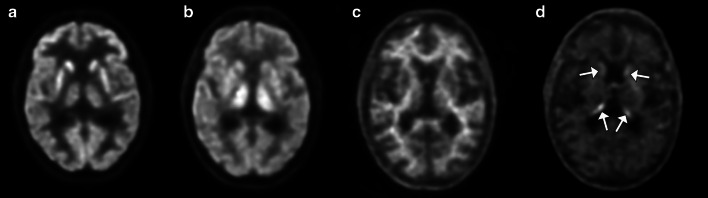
Normal patterns of cerebral uptake across multiple radiotracers. Fluorodeoxyglucose PET/CT demonstrates high-grade uptake in the cortical and deep gray matter which should be greater than cerebellum (a). Cerebral blood flow imaging with early-frame or dynamic amyloid agents shows a similar pattern to FDG (b). Non-specific low-grade white matter activity with absence of gray matter activity is the normal pattern of delayed amyloid PET agents (c). Normal distribution of tau agents is low-grade gray and white matter activity with absence of elevated neocortical uptake and a variable amount of off-target binding, commonly involving the basal ganglia and choroid plexuses (arrows in d)
